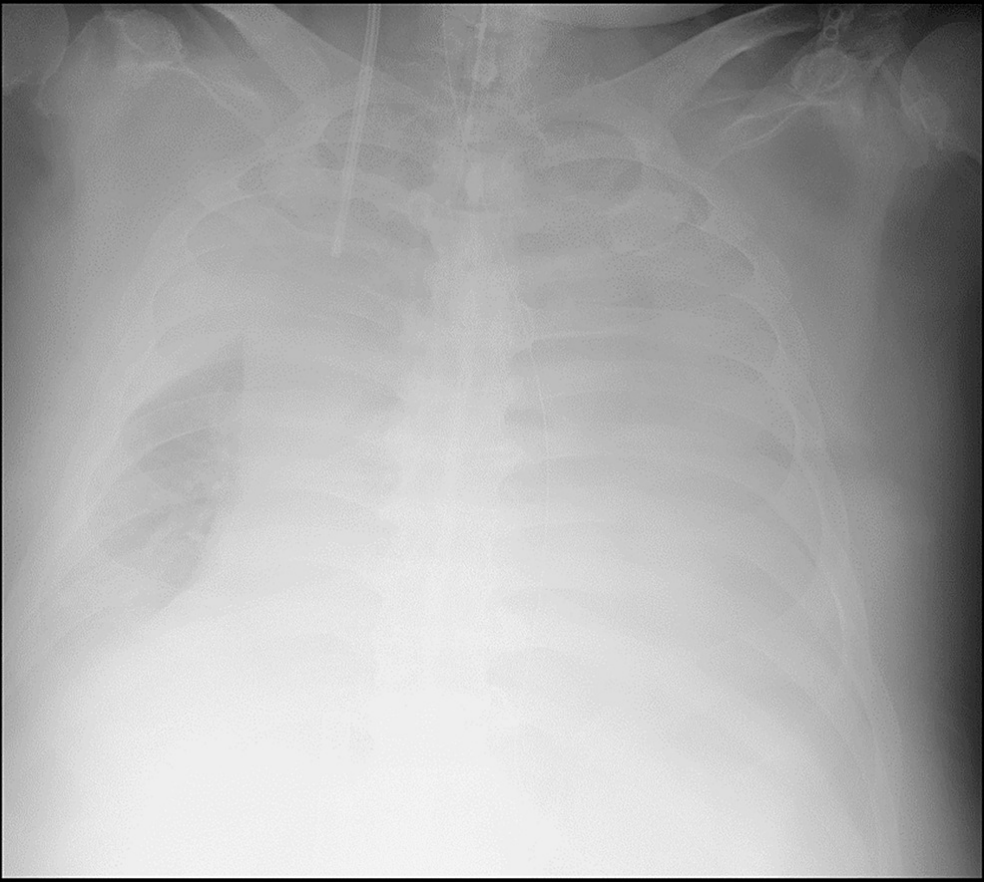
Chest X-ray on the 5th hospital day (before CNAP therapy). The image shows extensive bilateral atelectasis. CNAP: Continuous negative abdominal pressure
